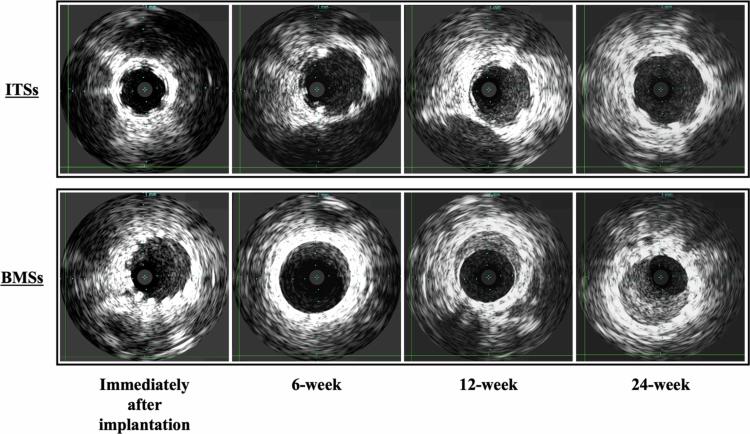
Representative intravascular ultrasonography images immediately after implantation and at 6-week, 12-week, and 24-week follow-ups. ITS, Igaki-Tamai stent; BMS, bare metal stent.
